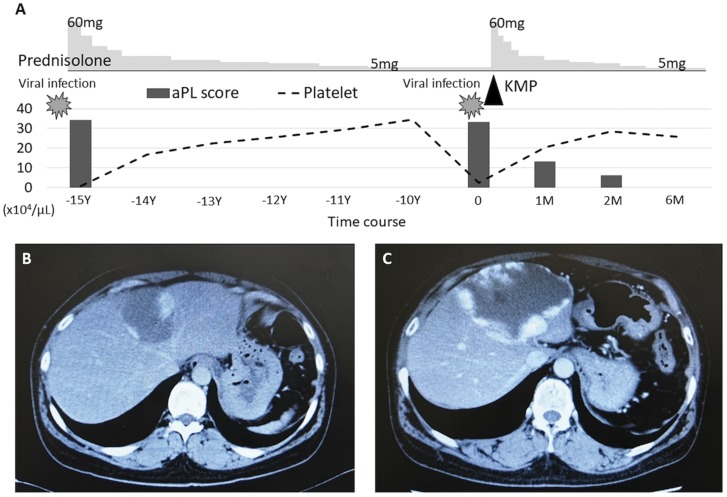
Clinical course and laboratory findings (A) The aPL score was calculated according to type of positive aPL test and their titers. Liver contrast-enhanced CT images in the first (B) or second (C) episode of thrombocytopenia. Peripheral enhancement of the hypoattenuating liver tumours indicates haemangioma. KMP: Kasabach–Merritt phenomenon.
